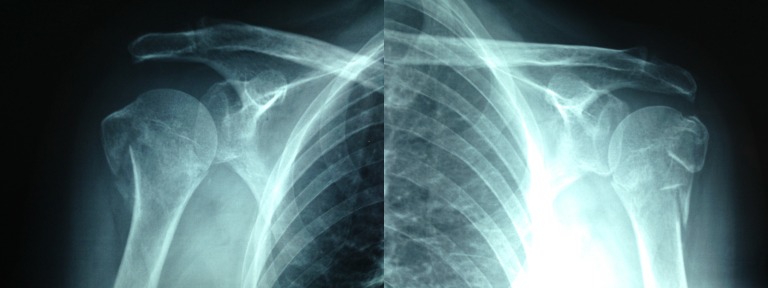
Simple x-rays AP bilateral views, week 3
